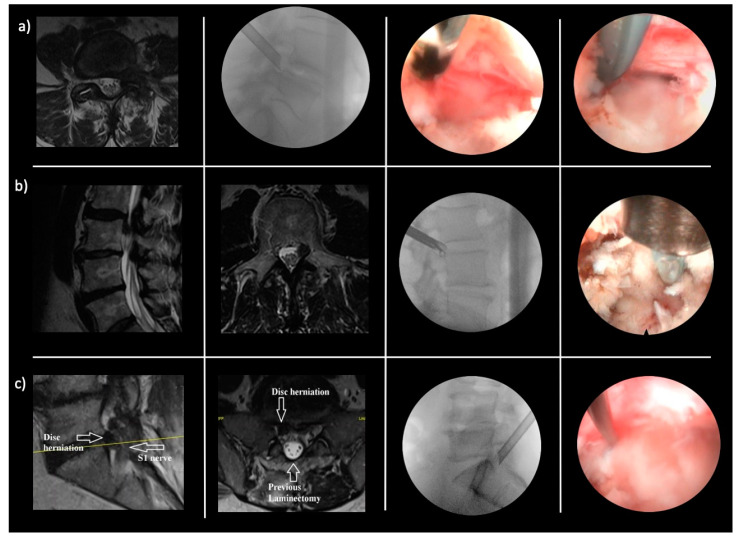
Common examples of pain generators missed by the routine lumbar MRI scan: (a) Inflamed nerve. Left to right: T2 axial MR image demonstrating the severe left L4–5 foraminal stenosis and foraminal disc herniation. Lateral fluoroscopic image of the beveled tubular retractor in the left L4–5 foramen. Endoscopic camera view of the inflamed left L5 traversing nerve root not demonstrated on MRI scan. Endoscopic camera view of the radiofrequency probe elevating the traversing left L5 never root and revealing the compressing disc fragment below. (b) Nerve tethering. Left to right: T2 sagittal MR image demonstrating the caudally extruded disc fragment behind the body of L3. T2 MR axial image of the left L2–3 caudally extruded disc fragment medial to the left L3 pedicle. Lateral fluoroscopic image of the bendable endoscopic grasper removing the caudally extruded disc fragment. Endoscopic camera view of the extruded disc fragment visible after removing the cranial portion of the L3 pedicle. A radio frequency probe is demonstrated above the L3 pedicle. The tethering of the nerve root by scar tissue was missed by the MRI scan. (c) Hypervascular scar. Left to right: T2 sagittal MR image demonstrating the L5-S1 disc herniation compressing the S1 nerve. T2 MR axial image demonstrating the L5-S1 disc herniation and the significant laminectomy defect. Lateral fluoroscopic images demonstrate the position of the beveled 7 mm tubular retractor in the right L5-S1 foramen. Endoscopic camera view of the endoscopic ball probe used to dissect the S1 nerve off the disc herniation. The MRI scan failed to show the severe hypervascular scar that had to be dissected and coagulated to release the S1 nerve and remove the herniated disc.
